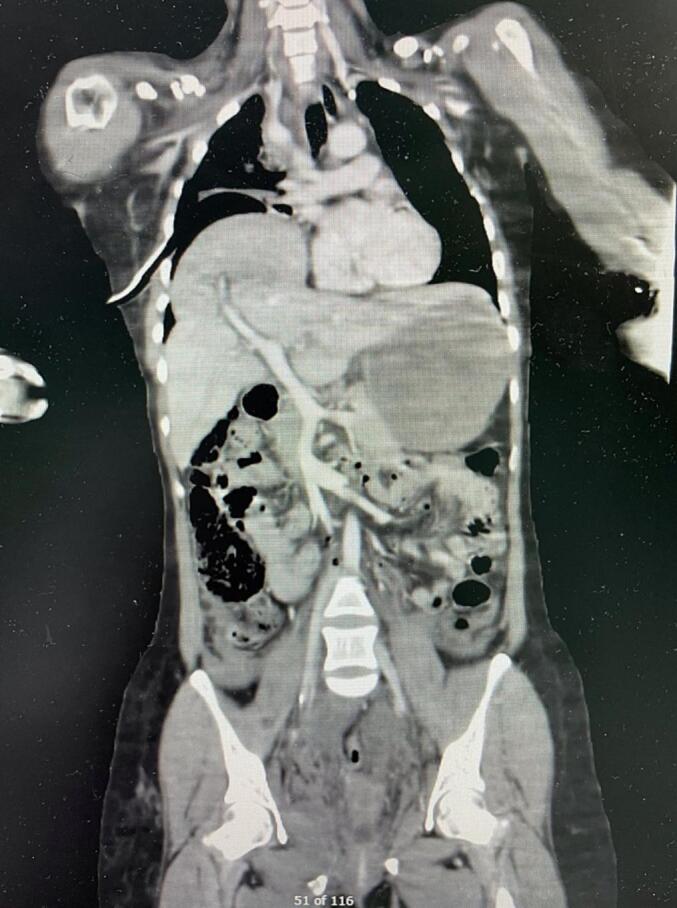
A CT scan with a coronal view demonstrates the right dome of the liver inside the chest with mediastinum shift.
